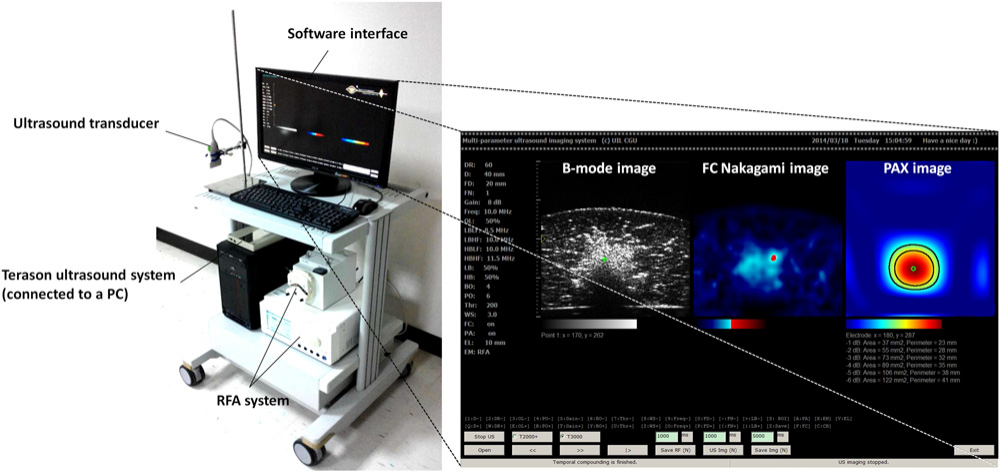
Real-time Nakagami imaging system for monitoring RFA. The proposed algorithm is integrated into a PC-based clinical ultrasound scanner (Terason ultrasound).
